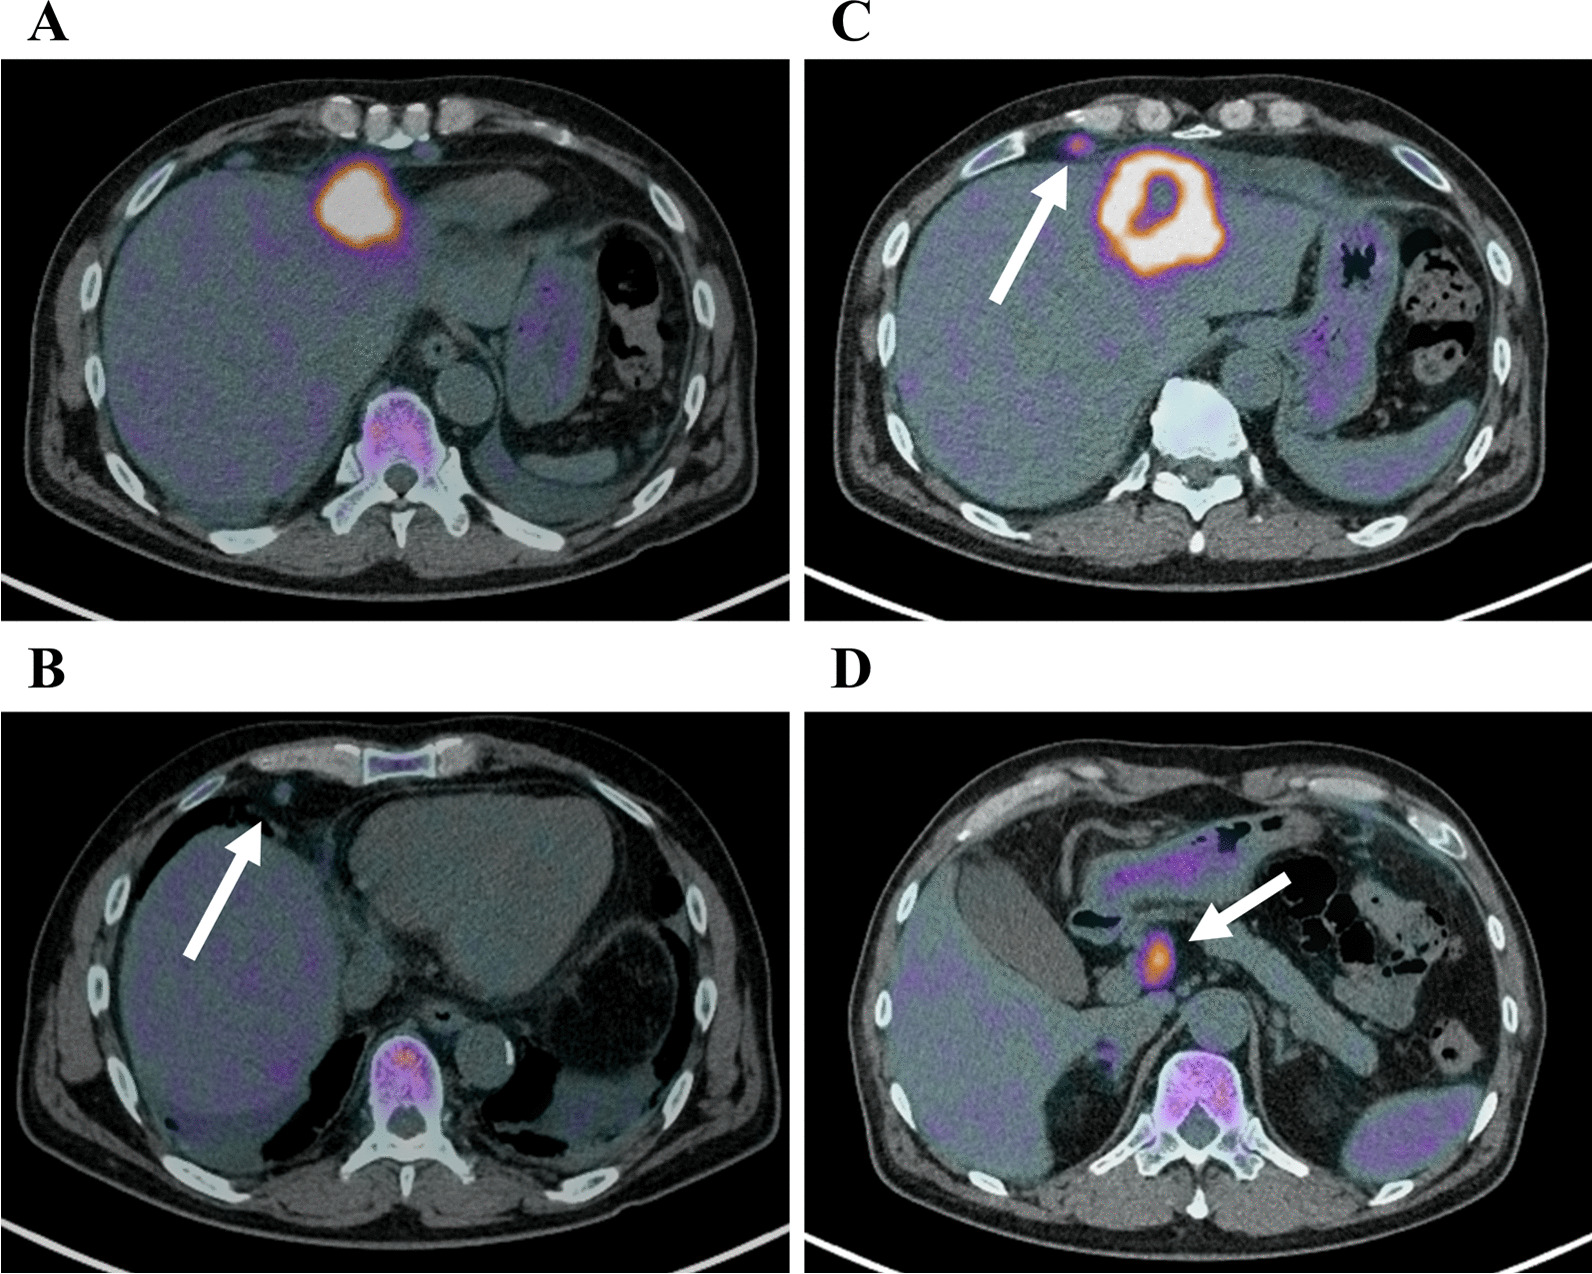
Abdominal fluorodeoxyglucose/positron emission tomography before systemic chemotherapy and transcatheter hepatic arterial injection. A Abdominal fluorodeoxyglucose/positron emission tomography shows a strong accumulation of fluorodeoxyglucose (FDG) in the tumor present in the left lobe of the liver. B, C Accumulation of FDG is observed in multiple lymph nodes around the heart (white arrows). D Accumulation of FDG is observed in the perihilar lymph node (white arrows)
